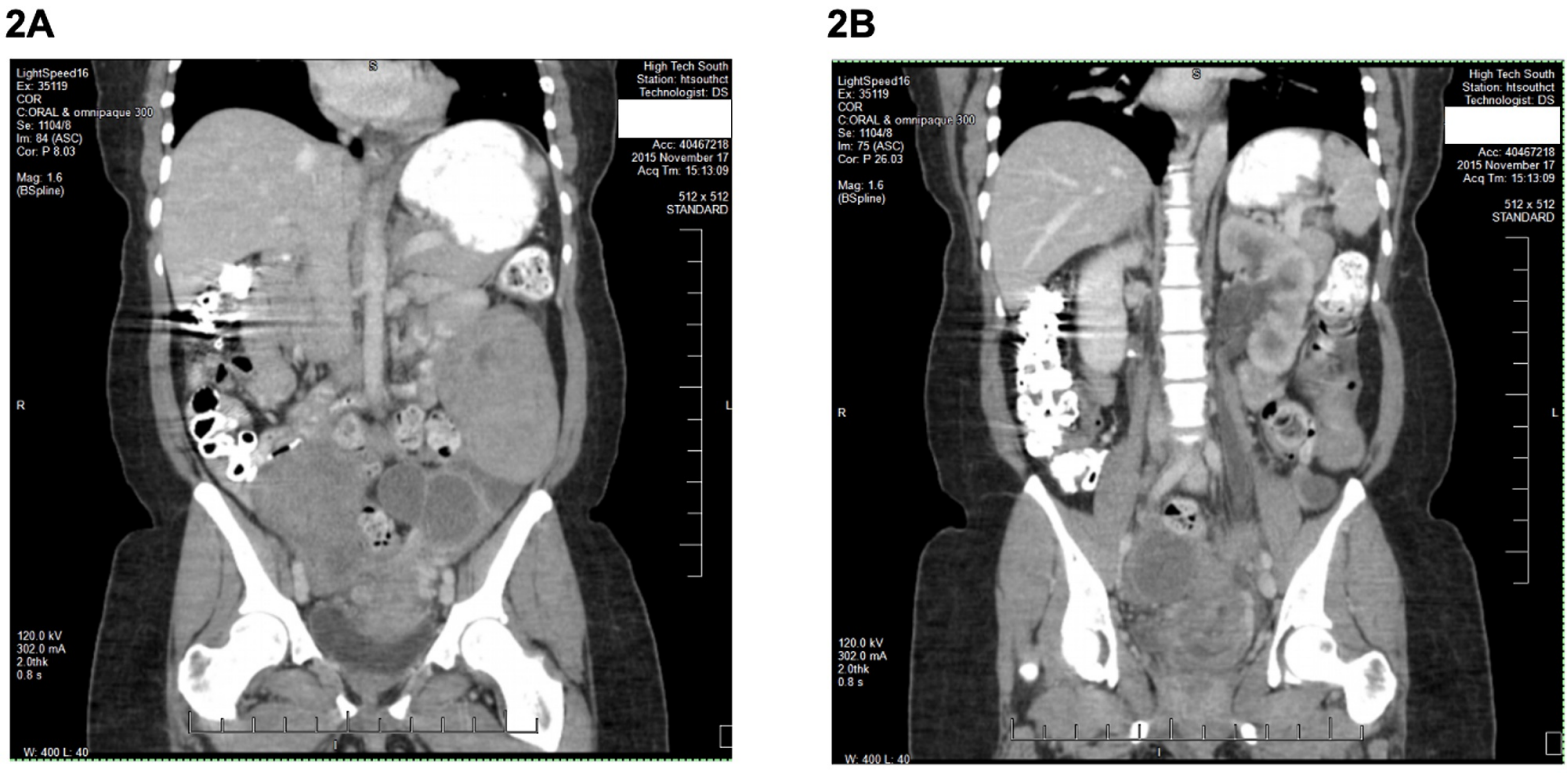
Pelvic CT showing an ovarian multiloculated cyst on the left measuring 8.8 x 6.8 cm (A), and another cystic process on the right measuring 5.1 x 4.1 cm (B).
